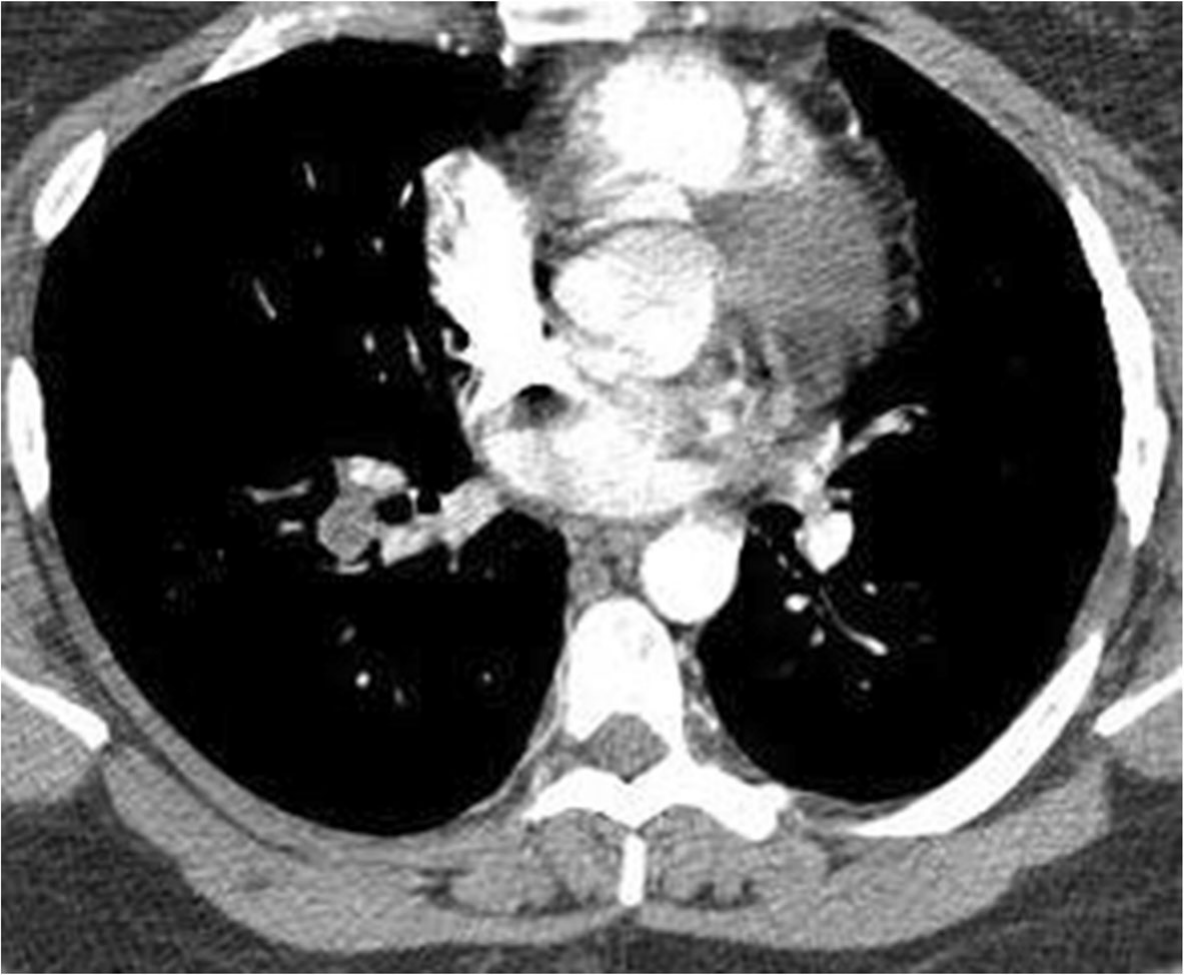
Computed tomography angiography of the chest with right-sided arterial emboli. Coronal section of this patient’s computed tomography angiography demonstrates a filling defect within the pulmonary vasculature, most likely representing emboli. At the time of this study, the patient was being treated with a novel oral anticoagulant (rivaroxaban)
